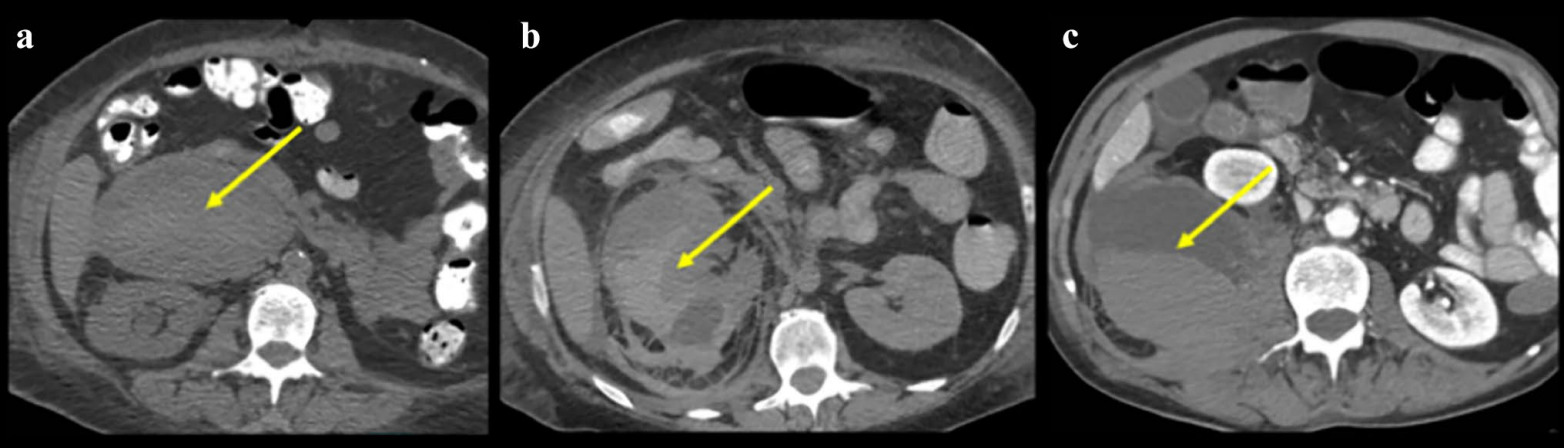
Cases depicting the a anterior pararenal space, b perirenal space, and c posterior pararenal space by collections and/or hematomas on axial CT sections. Pathology preferentially courses inferolaterally in the anterior pararenal space, dorsomedially to the lower pole in the perirenal space, and inferolaterally in the posterior pararenal space
